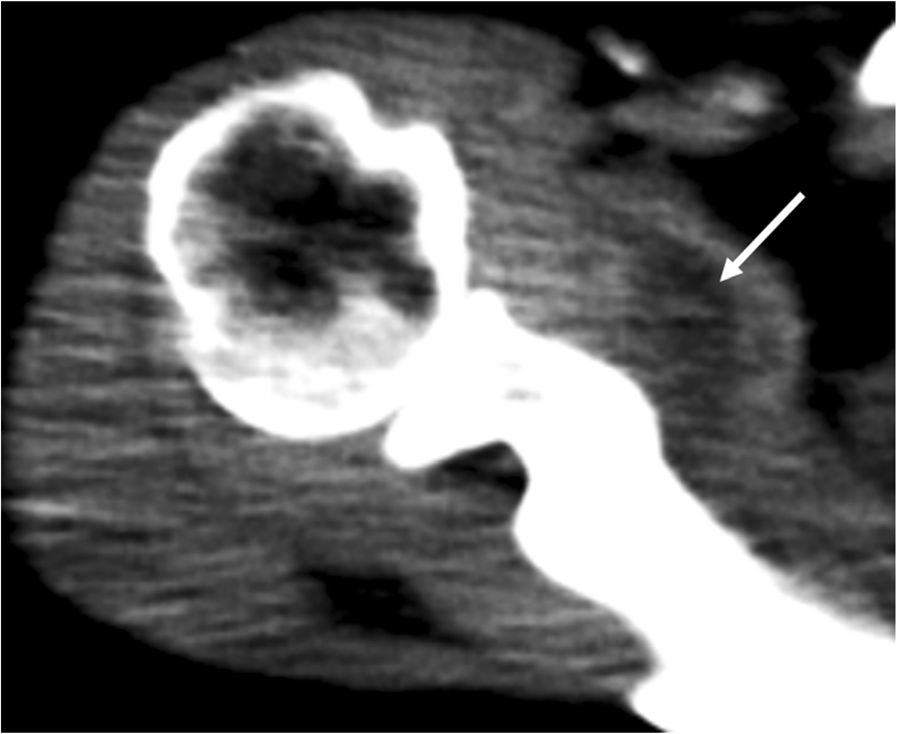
Contrast-enhanced computed tomography (CT) image performed at admission demonstrated abscess formation in the right subscapularis muscle (arrow)
